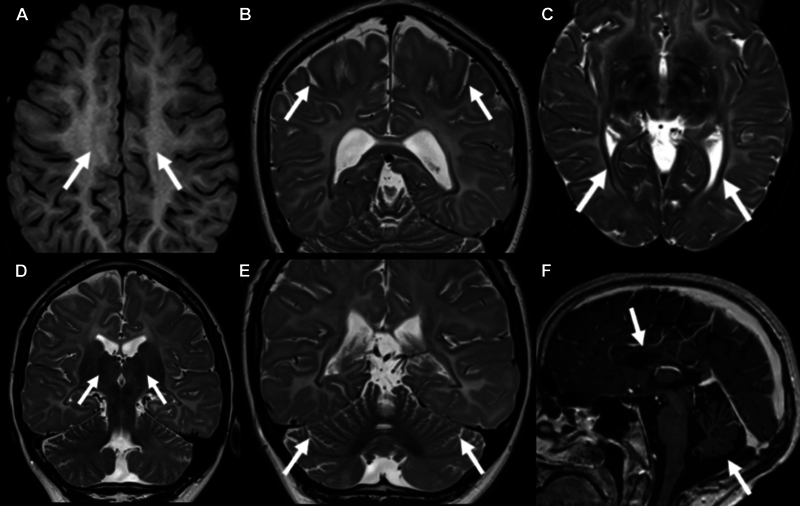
Magnetic resonance imaging (MRI) findings in POLR3-related leukodystrophies – diffuse hypomyelination, cerebellar atrophy, and thalamic T2 hypointensity. Axial T1-weighted (
